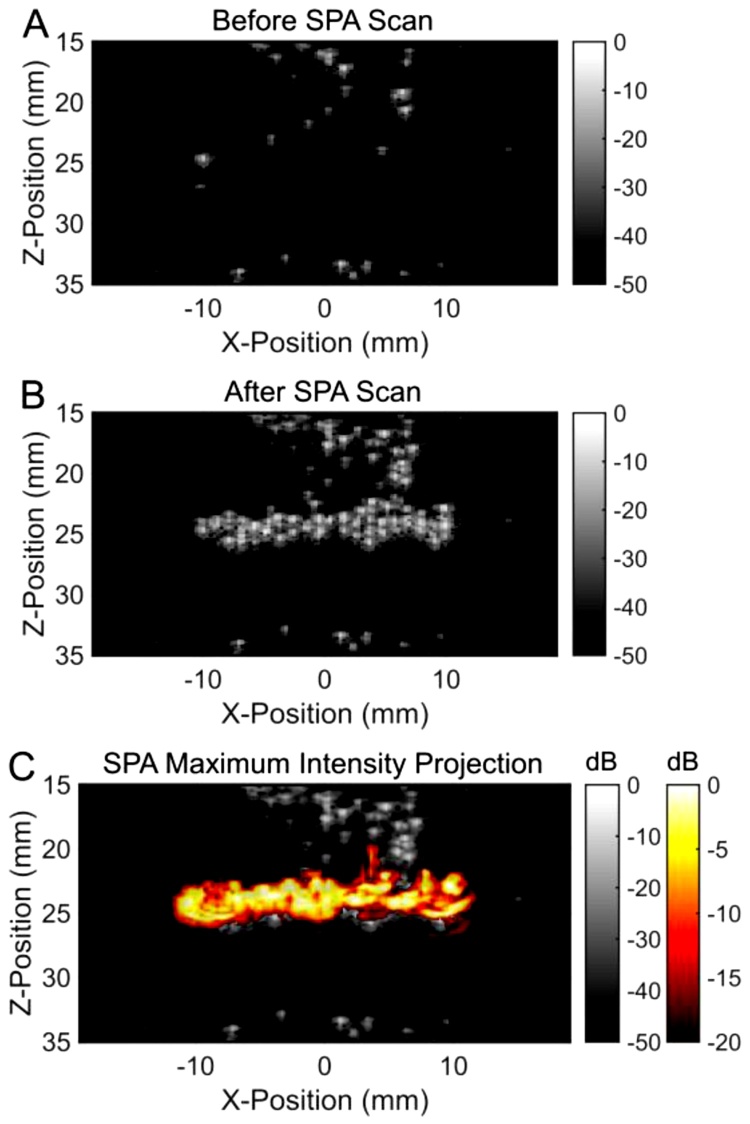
An example of irreversible SPA activation. B-mode ultrasound images (A) before and (B) after SPA activation of perfluorobutane (T_Boiling_ = −2 °C) droplets. (C) Maximum intensity projection of SPA contrast overlaid on the final ultrasound image. Due to the high volatility of perfluorobutane droplets, they did not return to the liquid phase after activation. This resulted in persisting ultrasound contrast seen in panel B.
